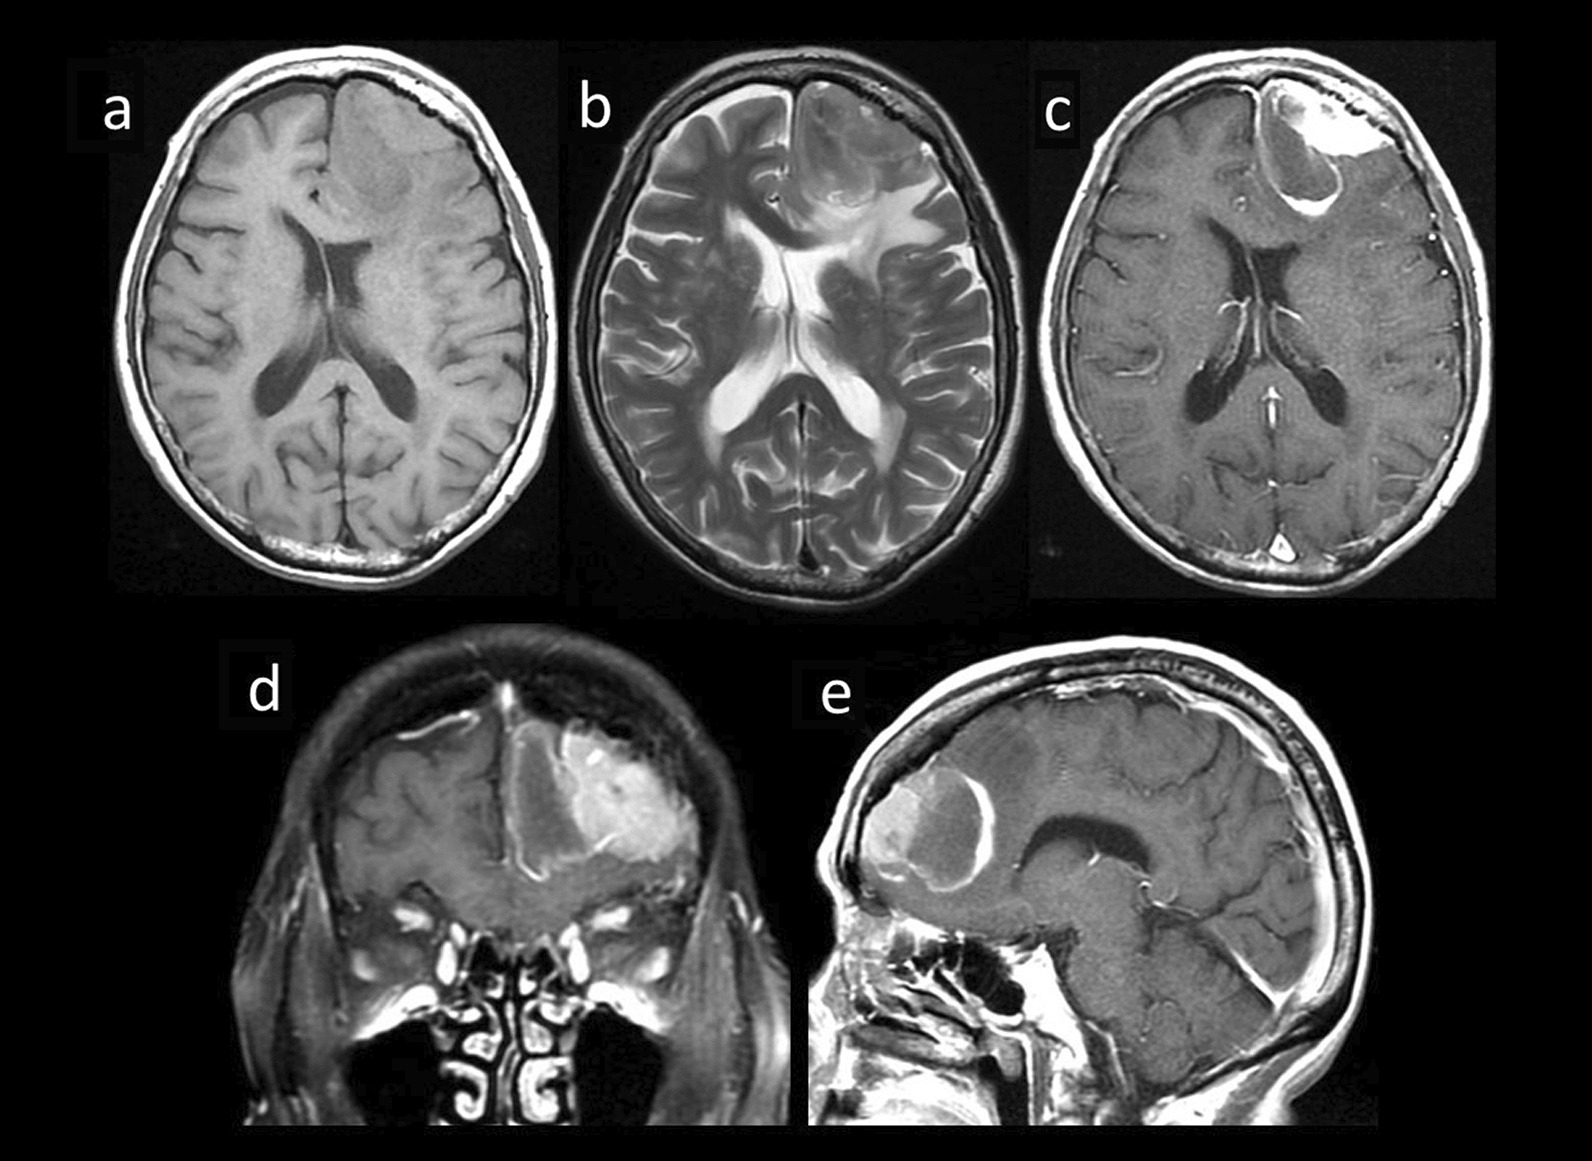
Magnetic resonance imaging 6 months later shows ring enhanced mass by the dural-based mass. a The axial T1-weighted image. b The axial T2-weighted image. c The axial contrast-enhanced image. d The coronal contrast-enhanced image. e The sagittal contrast-enhanced image
